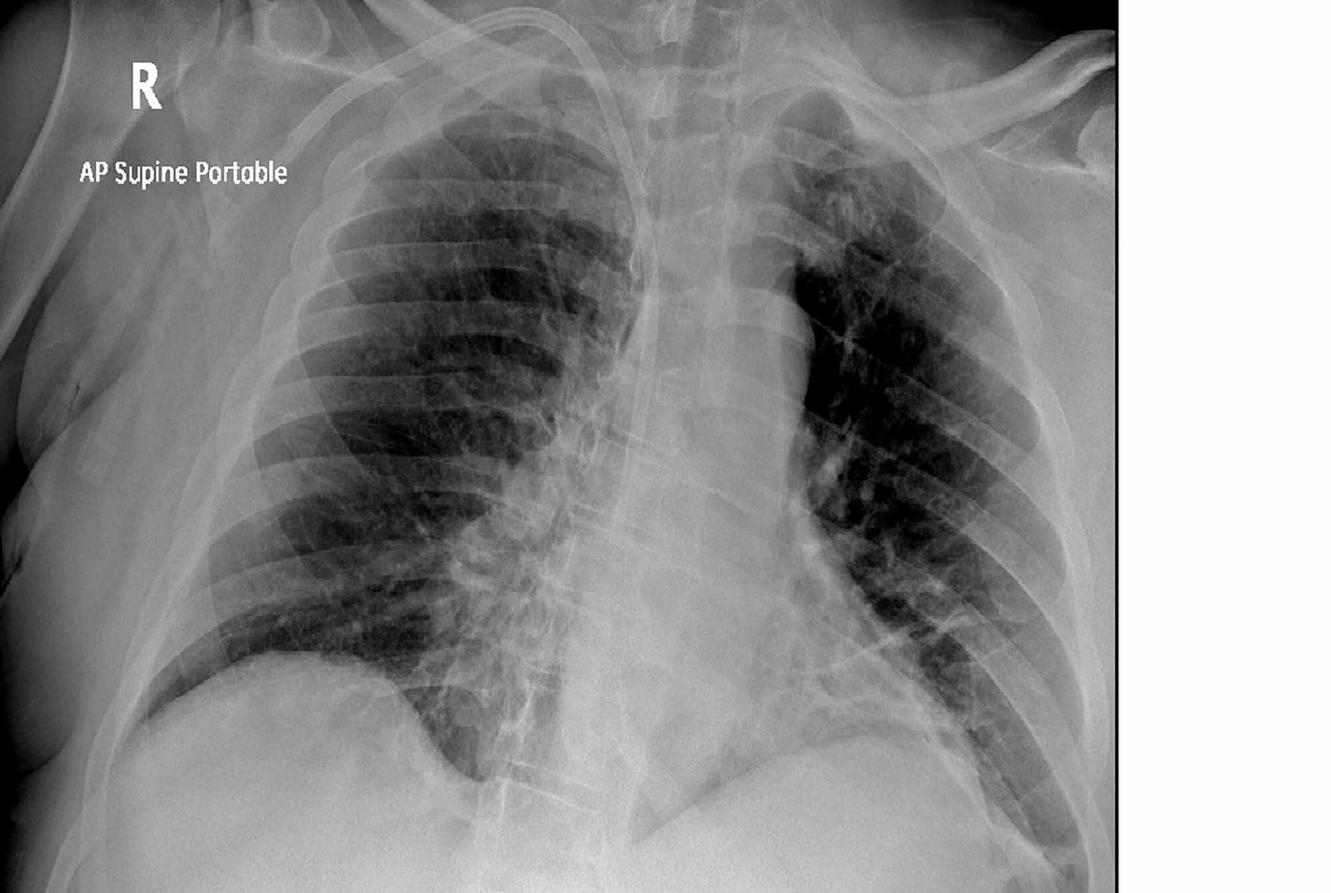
Chest X-ray showing improvement in infiltrates after treatment with azithromycin
